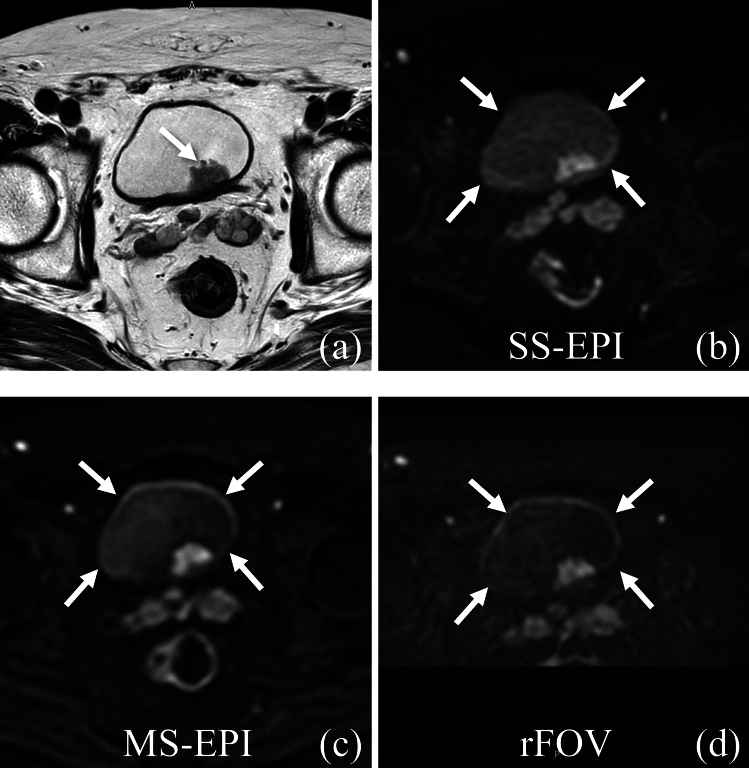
Qualitative evaluation of bladder wall clarity in a 71 year-old man with non-muscle invasive bladder cancer. a T2-weighted imaging shows a broad-based tumor (white arrow) at the posterior bladder wall. b Single-shot echo planar imaging diffusion weighted imaging (SS-EPI DWI). The bladder wall (white arrows) is poorly visualized. c Multi-shot echo planar imaging diffusion weighted imaging (MS-EPI DWI). Visualization of the bladder wall (white arrows) is excellent compared to SS-EPI DWI and reduced field-of-view diffusion weighted imaging (rFOV DWI). d rFOV DWI. The bladder wall (white arrows) is moderately delineated with blurred margin but is better than SS-EPI DWI
